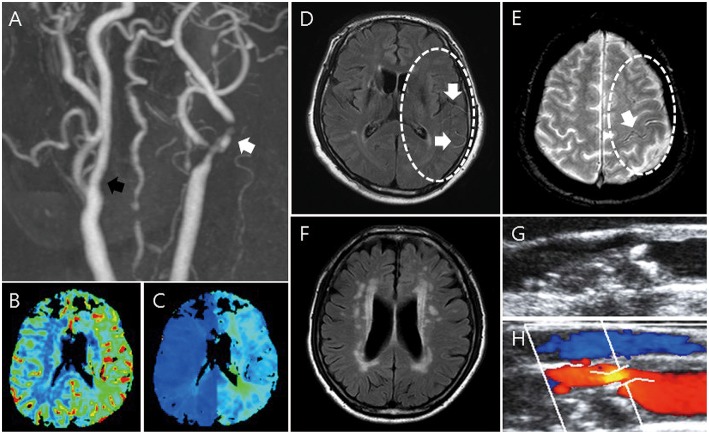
Imaging parameters: MRI, CTA/MRA, PWI, and carotid duplex sonography. MRA shows stenosis of the proximal internal carotid artery (A, white arrow) and contralateral carotid artery stenosis (A, black arrow). In PWI, increased CBV (B) and delayed TTP (C) are shown. FLAIR shows HVS in FLAIR (D) and PWMH/DWMH (F). GRE shows HV in GRE (E). Through duplex carotid sonography, plaque characteristics such as calcification, ulceration, echolucent, and stenosis are shown (G,H).
